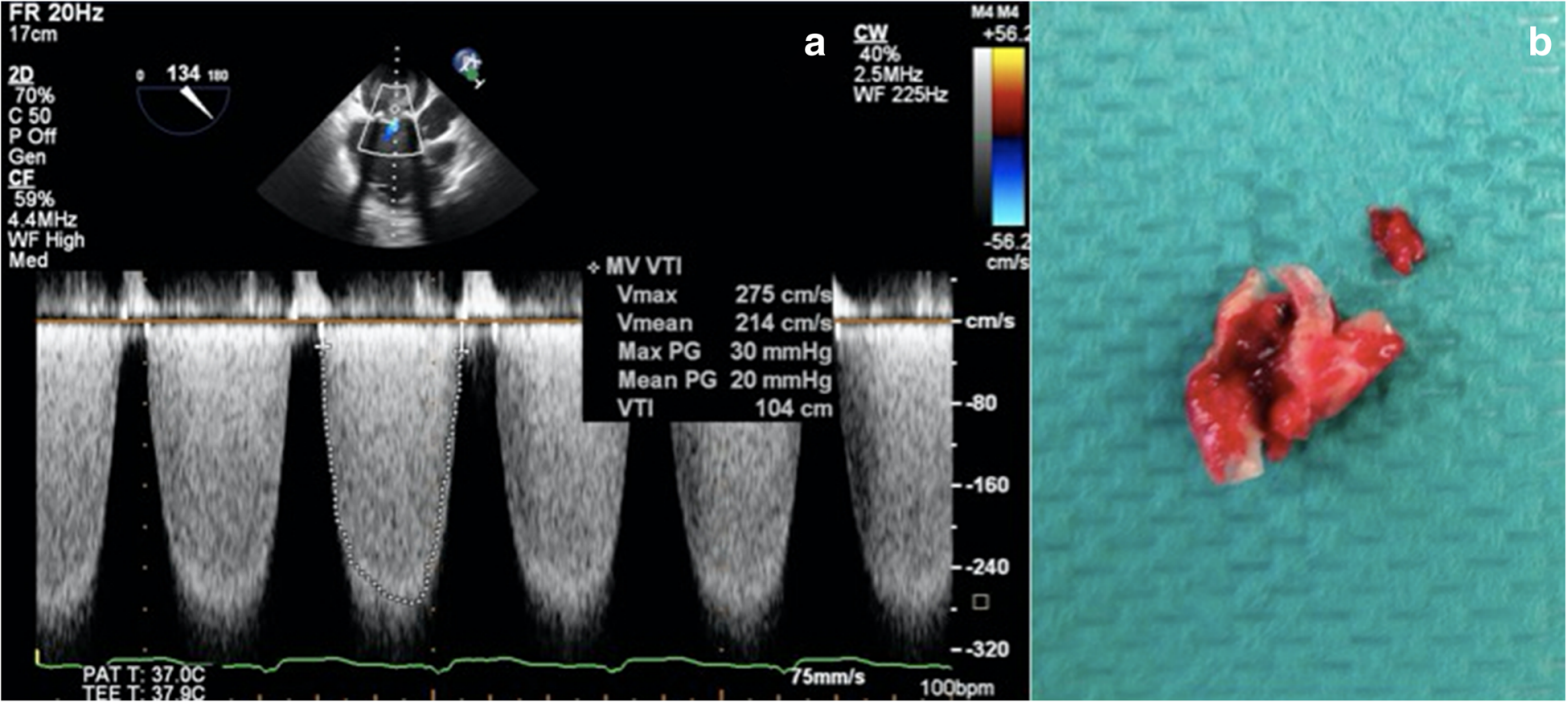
a Mid-esophageal long-axis view with continuous-flow Doppler showing a mean mitral pressure gradient of 20 mmHg, which suggests severe mitral stenosis. b Organized thrombus adhered to the bioprosthetic mitral valve
